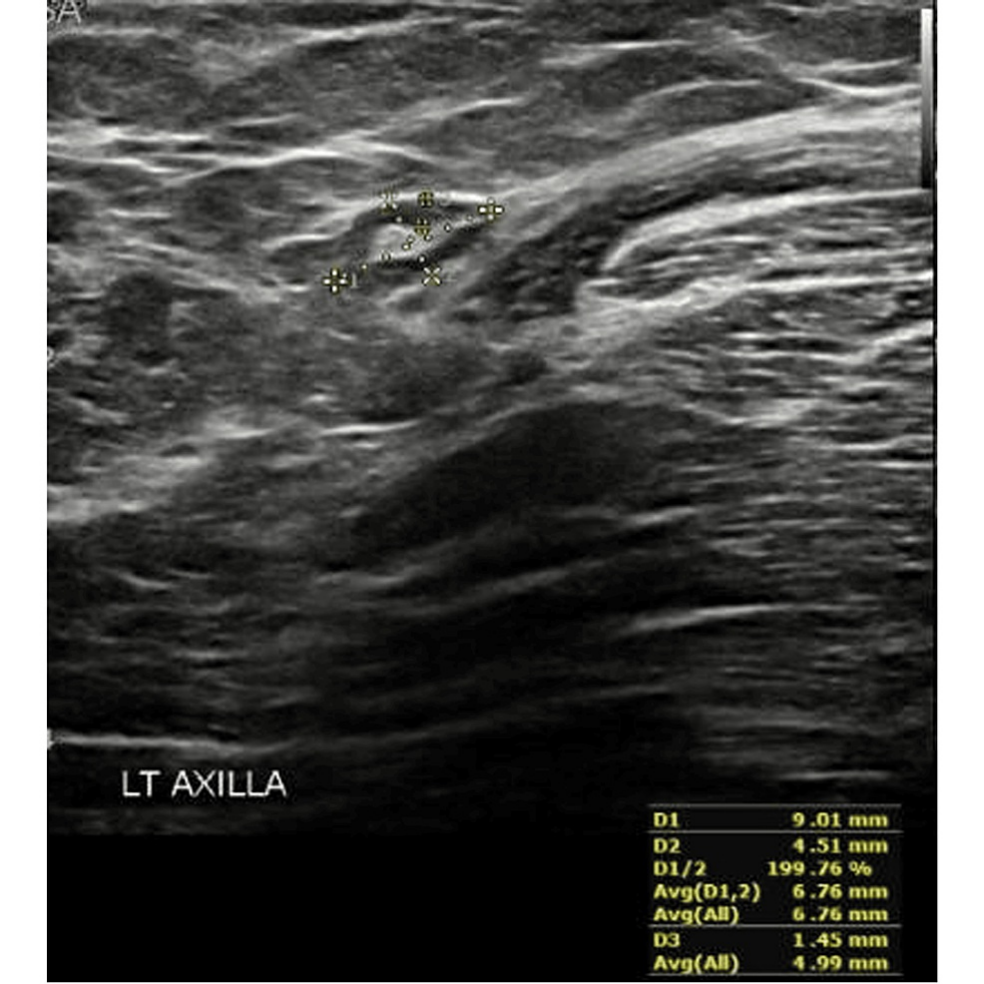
Ultrasound image showing a reactive axillary lymph node - Case B This figure was obtained from the KFUH system (author's own).
